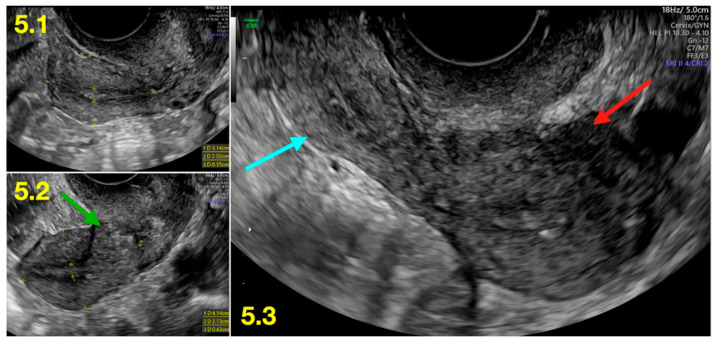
Transvaginal ultrasonography images at 6 months after cesarean section. (5.1) Right uterus, distance 1 (1D on image)—uterus length 3.14 cm, distance 2 (2D on image) uterus height 2.02 cm, distance 3 (3D on image)—endometrial thickness 0.35 cm. (5.2) Left uterus with a healed cesarean section scar (green arrow), distance 1 (1D on image)—uterus length 3.14 cm, distance 2 (2D on image) uterus height 2.02 cm, distance 3 (3D on image)—endometrial thickness 0.35 cm. (5.3) A normal post-pregnancy involution of uterus didelphys (blue arrow right uterus, red arrow left uterus).
